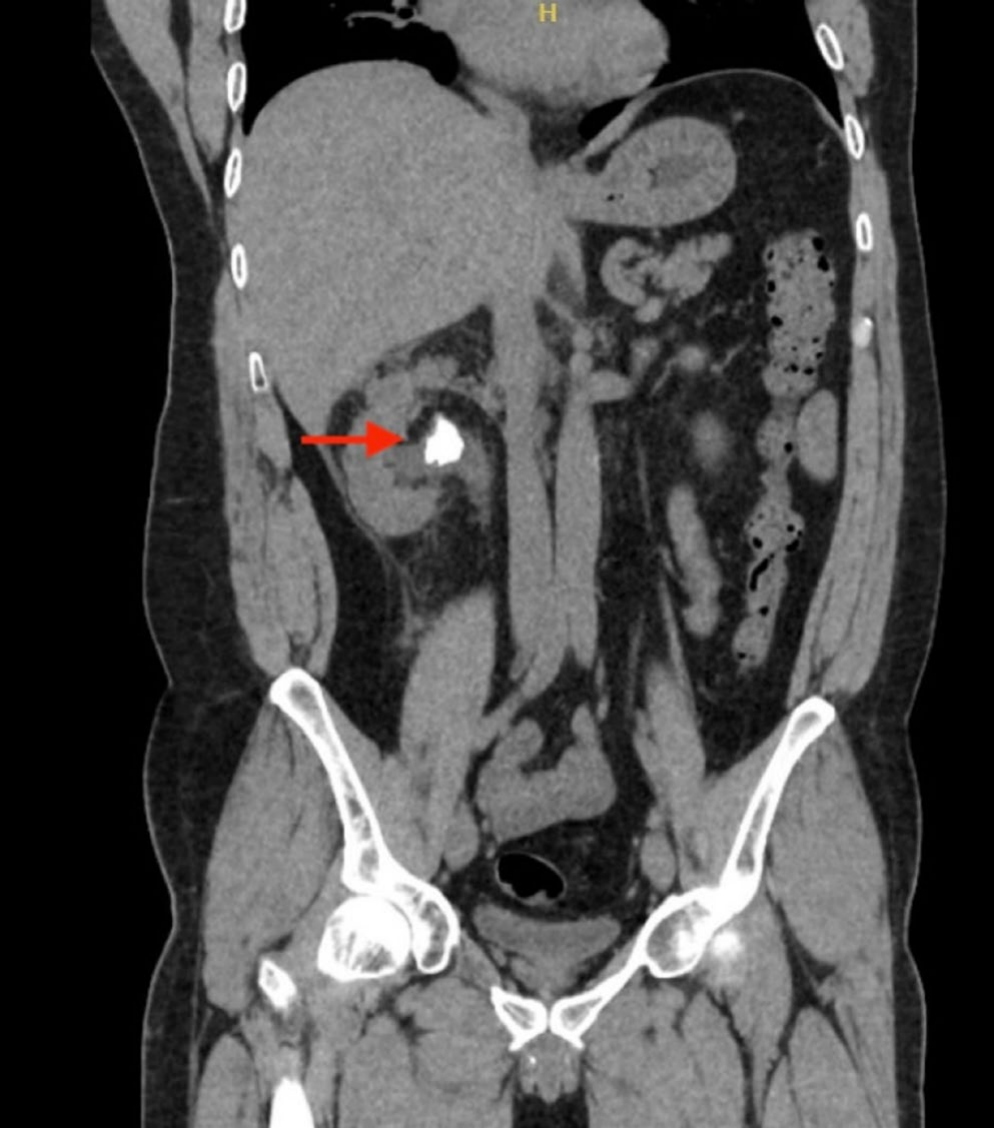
Coronal view of abdominal CT scan demonstrating a 2 cm calculus in the right renal pelvis.
